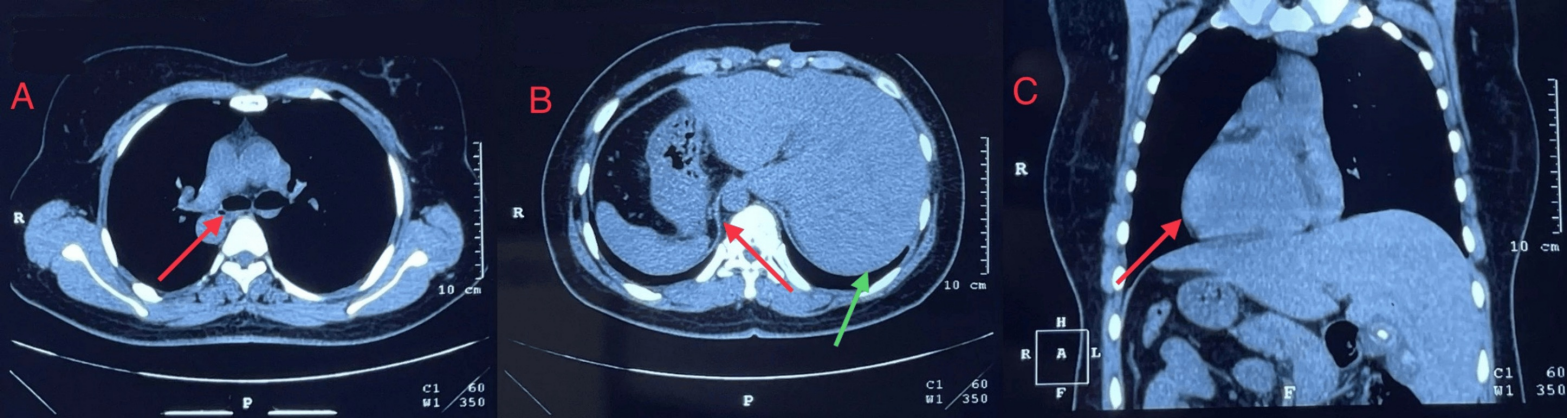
Non-contrast computed tomography (NCCT) scan of the thorax and upper abdomen. NCCT of the thorax and upper abdomen in panel A (red arrow) shows enlarged bronchi with thickened walls, consistent with bronchiectasis. The red arrow in panel B shows a hypodense gas shadow (gastric bubble) appearing on the right, while the green arrow in panel B shows liver parenchyma on the left, confirming situs inversus. Panel C shows the liver on the left and stomach gas shadow on the right, with the red arrow shows cardiac silhouette on the right, further confirming situs inversus totalis.
